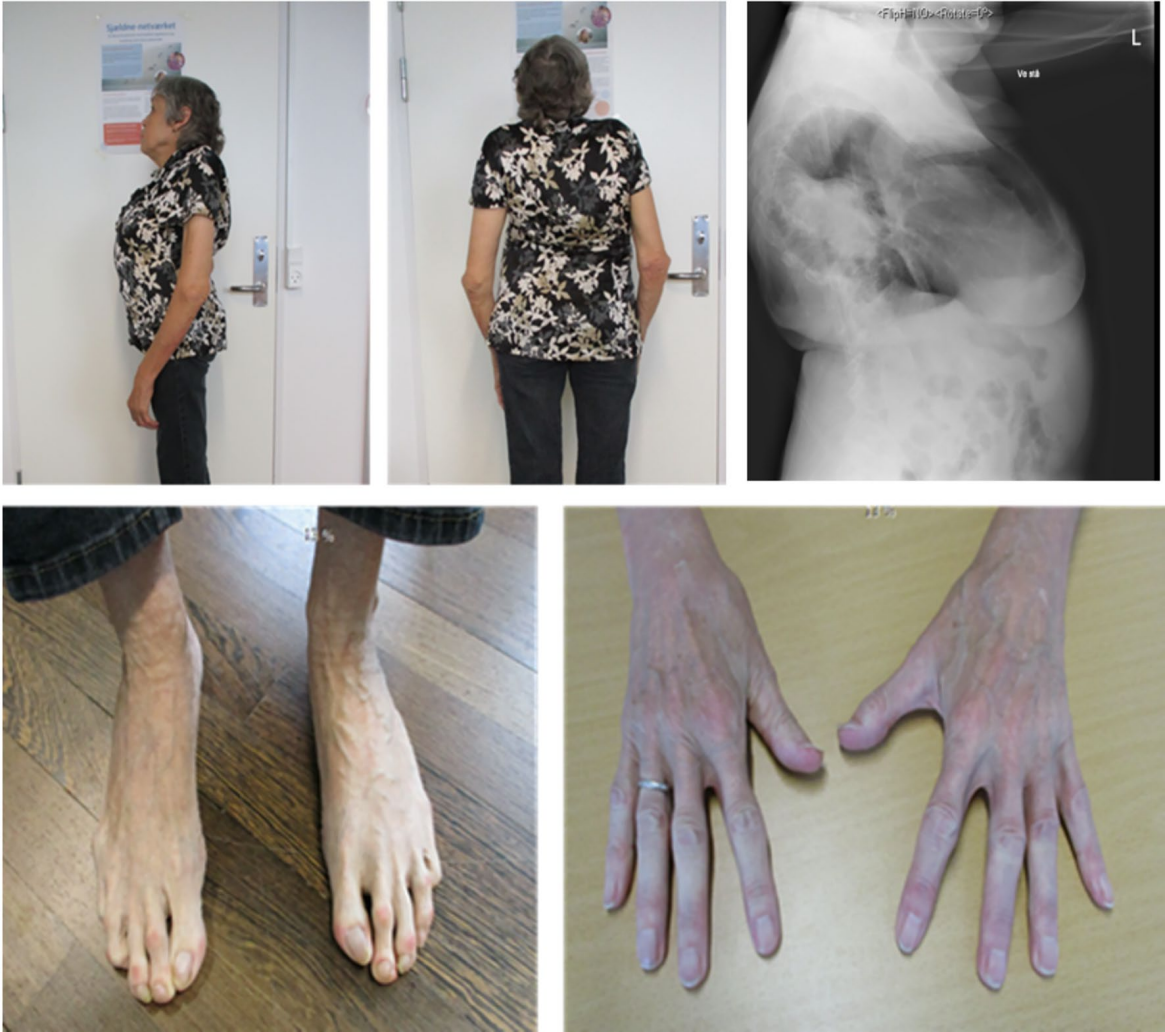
Subject 1. (A–B) The patient was clinically known to have kyphoscoliosis, pes planus, pectus carinatum, spatulate thumb, and hypermobility. (C) Radiographic lateral projection showing a chest X-ray). (D–E) Both hands and feet appeared elongated
